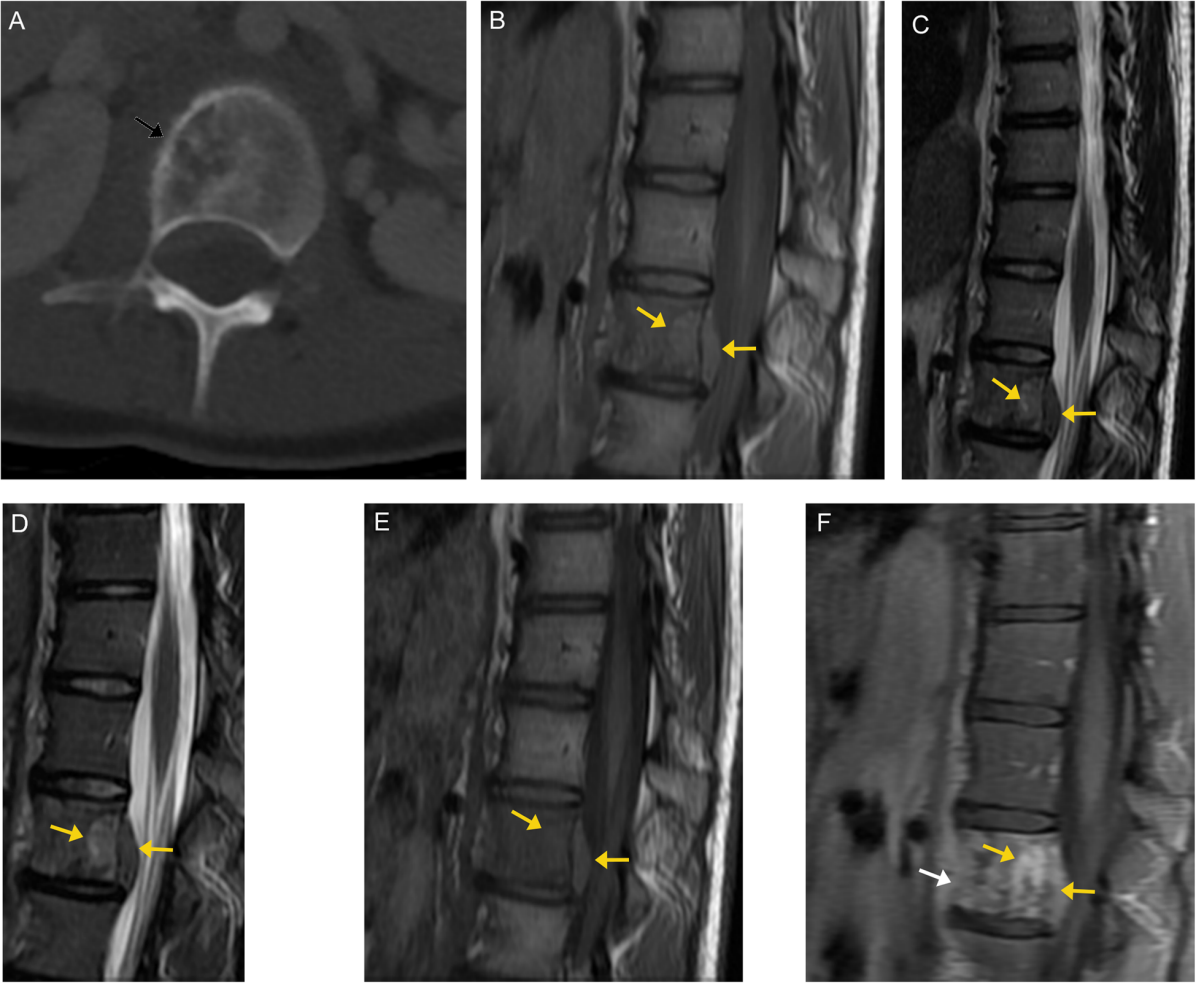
Ewing’s sarcoma. Axial CT image in the bone window (a) demonstrates an ill-defined lesion with destructive changes of the right vertebral body. The bone cortex has a “smudged” appearance (black arrow) due to perforation of tumor through the cortex. Sagittal proton-density image (b) demonstrates the ill-defined tumor as abnormal marrow signal with epidural extension (orange arrows). Sagittal T2-weighted (c) and T2 fat-saturated (d) images demonstrate variable signal within the lesion (orange arrows). This lesion demonstrates low to intermiediate T1 signal (e, orange arrows). On T1-weighted post-contrast imaging (f), there is heterogenous enhancement of the tumor (orange arrows), which is difficult to distinguish from the enhancing peritumoral edema (white arrow)
